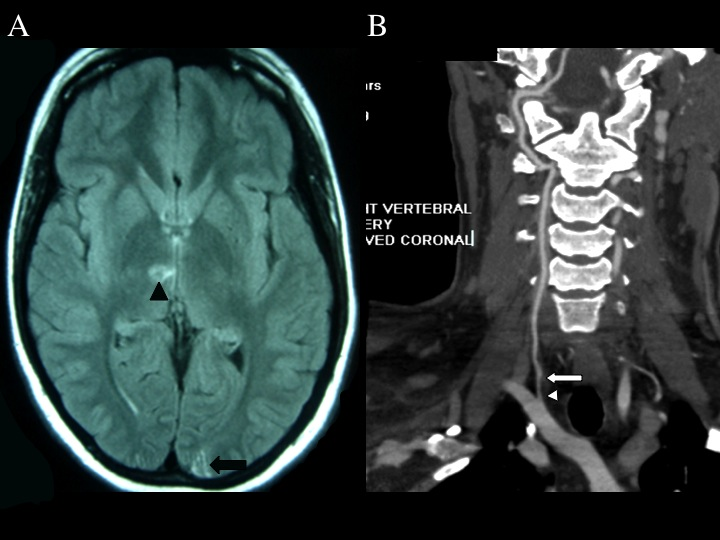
Diagnostic imaging results. (A) Magnetic Resonance Imaging Axial T2 FLAIR section showing a left occipital infarct (arrow) and also a right paramedian thalamic infarct (arrowhead). (B) Coronal view of the right vertebral artery on CT angiogram showing thinning of the artery (arrowhead) and shadowing of the dissected section of the vertebral artery (arrows).
